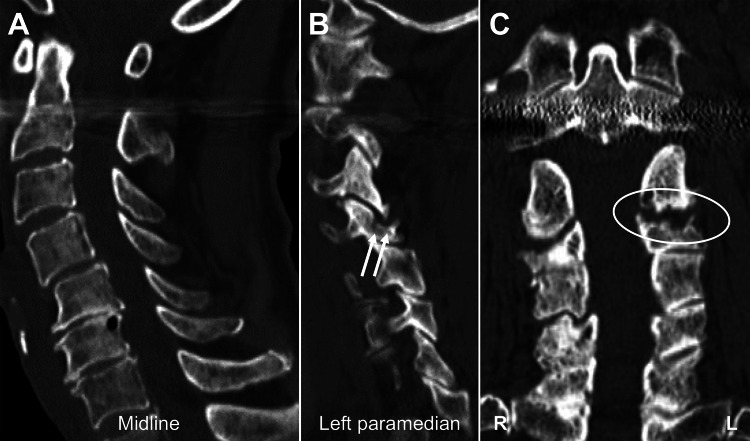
Preoperative cervical computed tomography images. (A) Midline sagittal image demonstrating subaxial spondylotic changes without spinal canal stenosis or spondylolisthesis at the C3-4 level. (B) Left paramedian sagittal image demonstrating a damaged superior articular surface of the left C4 facet joint (arrows). (C) Coronal image demonstrating erosion of the articular surface (circle).
